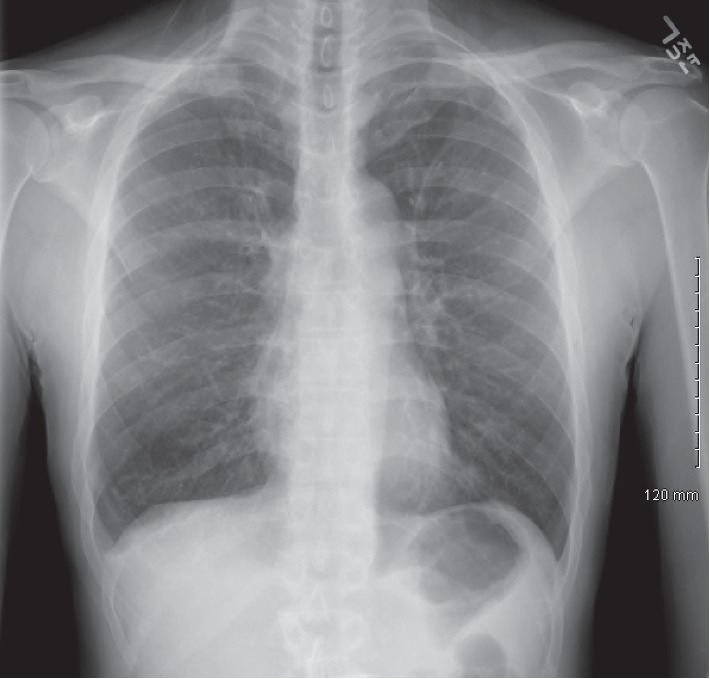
Posterior-anterior chest X-ray revealing hyperinflation, flattened diaphragm, and pleural thickening.
